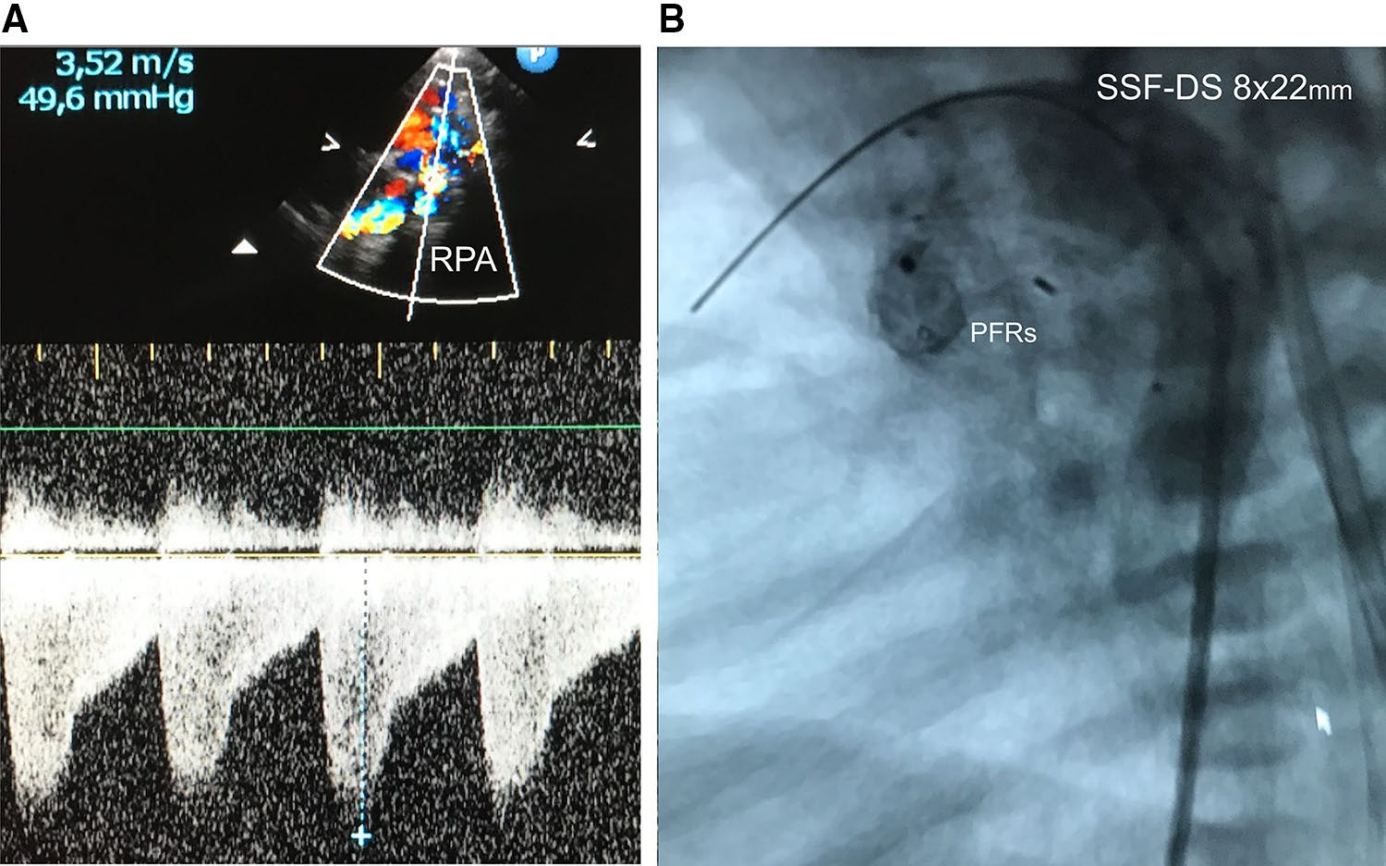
a, b Two-D- and color-Doppler echocardiographic short axis plane depicts the right pulmonary artery (RPA) and the belonging continuous wave (CW) Doppler tracing pulmonary systolic-diastolic flow profile representing an effective PFR (pulmonary flow restrictor, a lateral 90° angiography shows the final right-to-left shunt across the stented arterial duct using a SinusSuperFlex-DS stent (SSF-DS) and PFRs within the pulmonary artery branches b)
